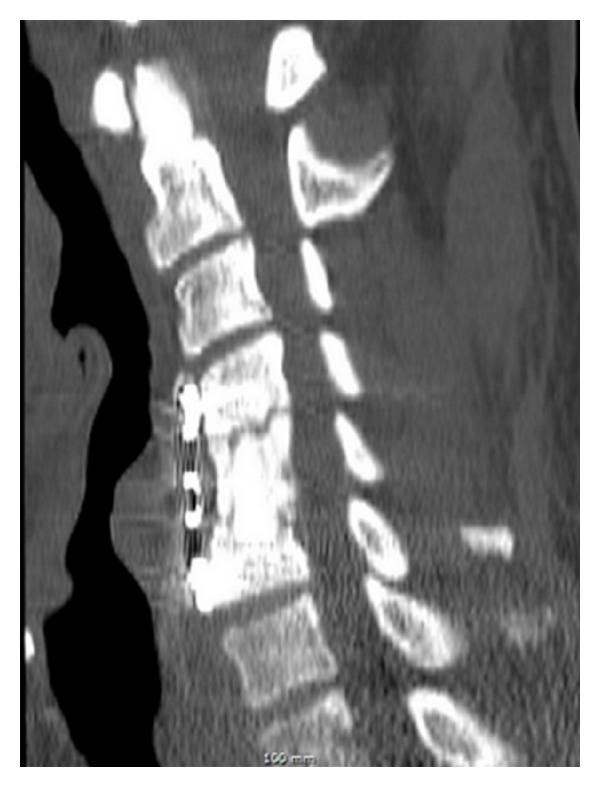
Sagittal CT reconstruction at the latest followup shows graft consolidation confirming fusion.
